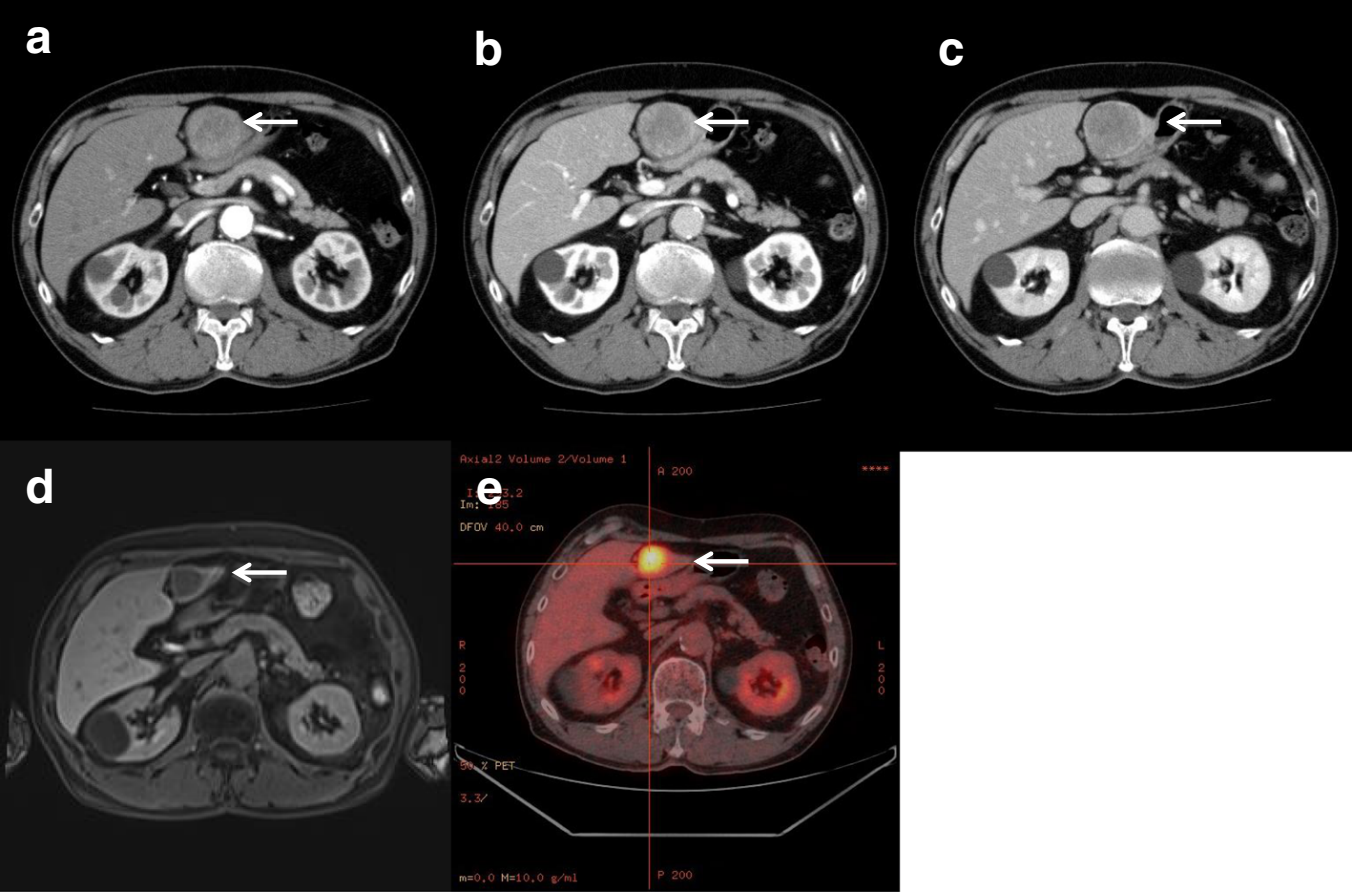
a–c Liver dynamic computed tomography (CT). a Arterial phase; b Portal phase; c Delayed phase. The tumor shows low signal intensity, with an unclear border in each phase (arrows). d Gd-EOB-DTPA-MRI shows tumor nodules in the liver and a low-intensity and hypo-vascular tumor in segment 3 (arrow). e Positron emission tomography-CT. The SUV max of the tumor in S3 of the liver is 10 (arrow)
